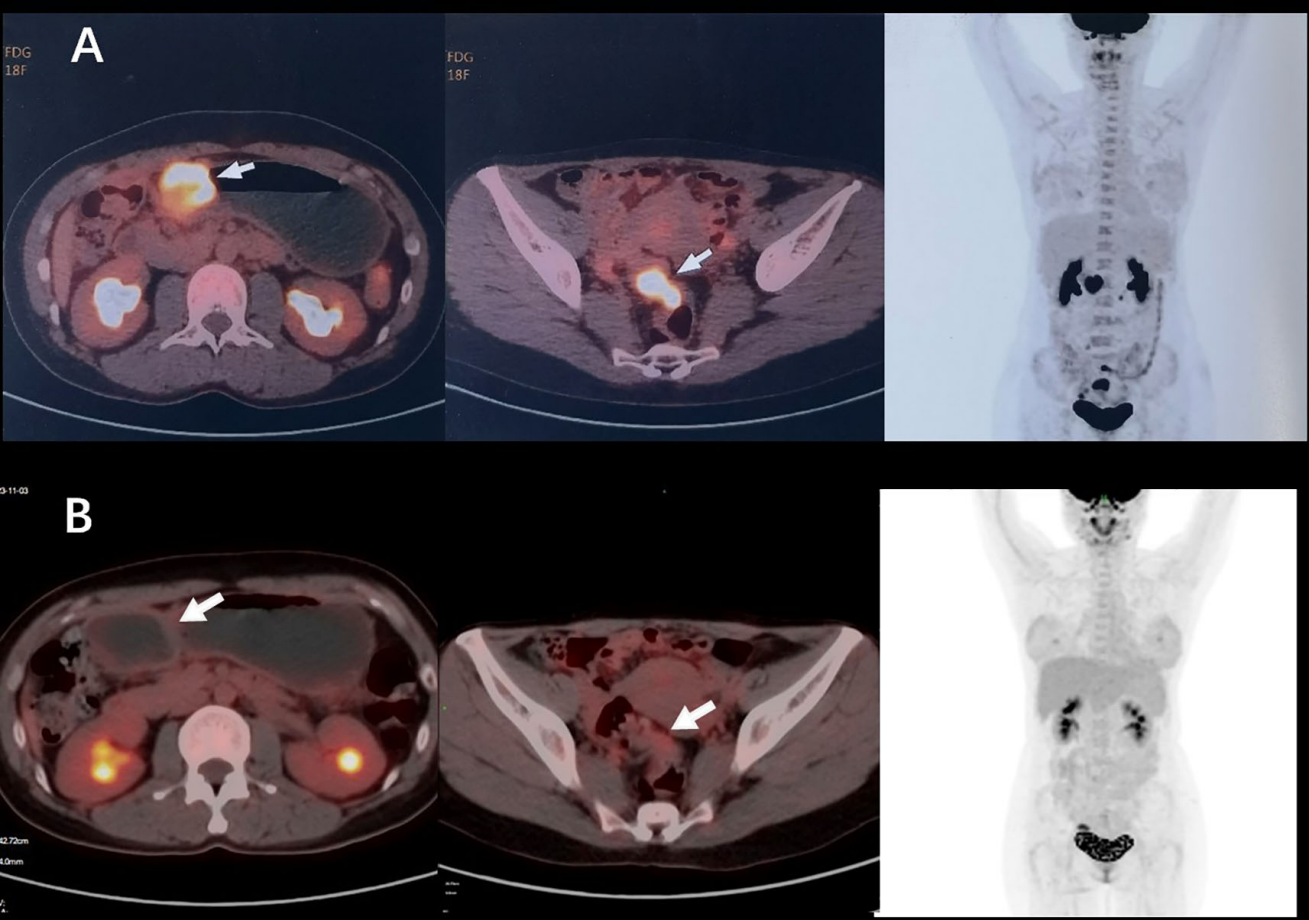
(A) Before systemic therapy, PET-CT showed multiple suspicious malignant lesions located at gastric antrum (SUVmax 8.0), rectosigmoid junction (SUVmax 9.4), and peritoneum without hypermetabolic activity. (B) After the treatment of nivolumab plus XELOX, PET-CT showed a residual lesion only at the rectosigmoid junction (SUVmax 3.1), and no hypermetabolic activity in the gastric region.
